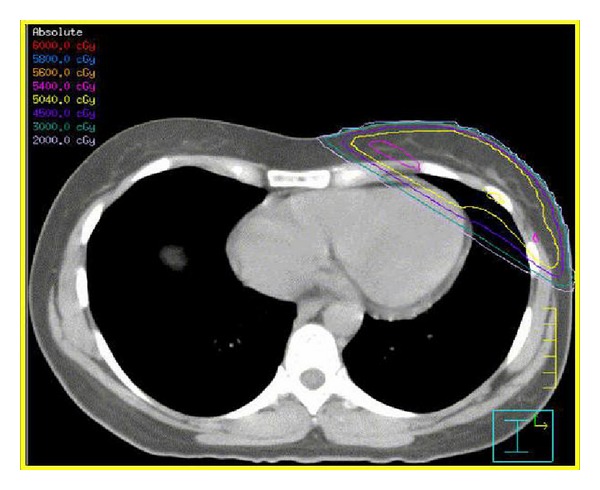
Radiation therapy planning CT with isodose distributions.
